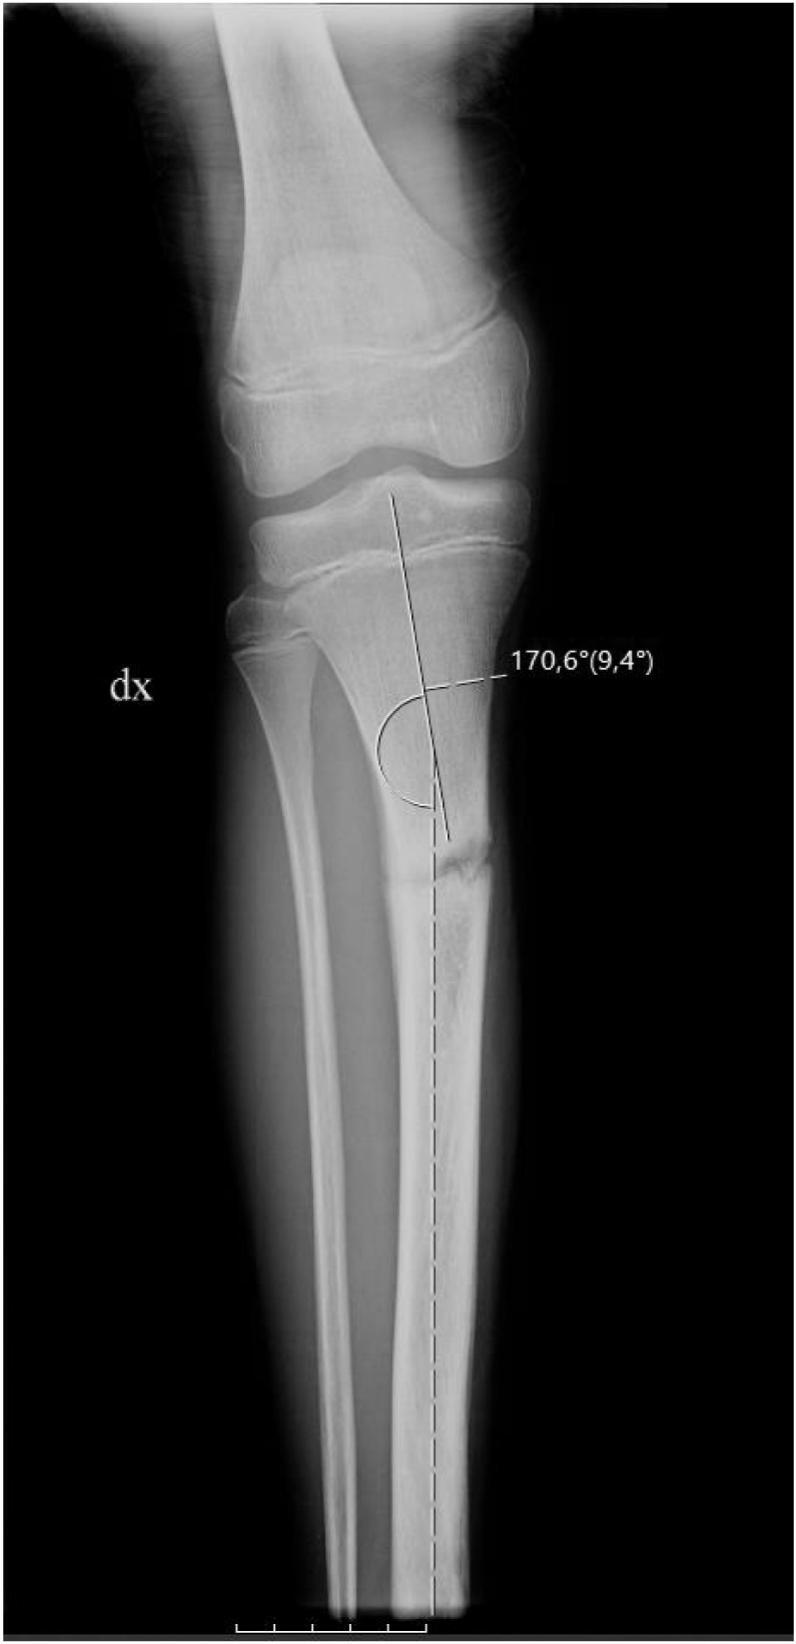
Local X-ray of the right leg 1 year after trauma.
